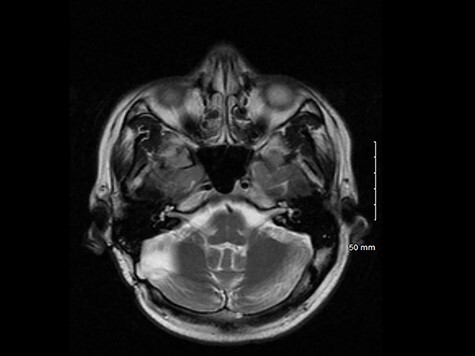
T2 weighted MRI axial image showing right cerebellar gliosis related to a previous surgery.
